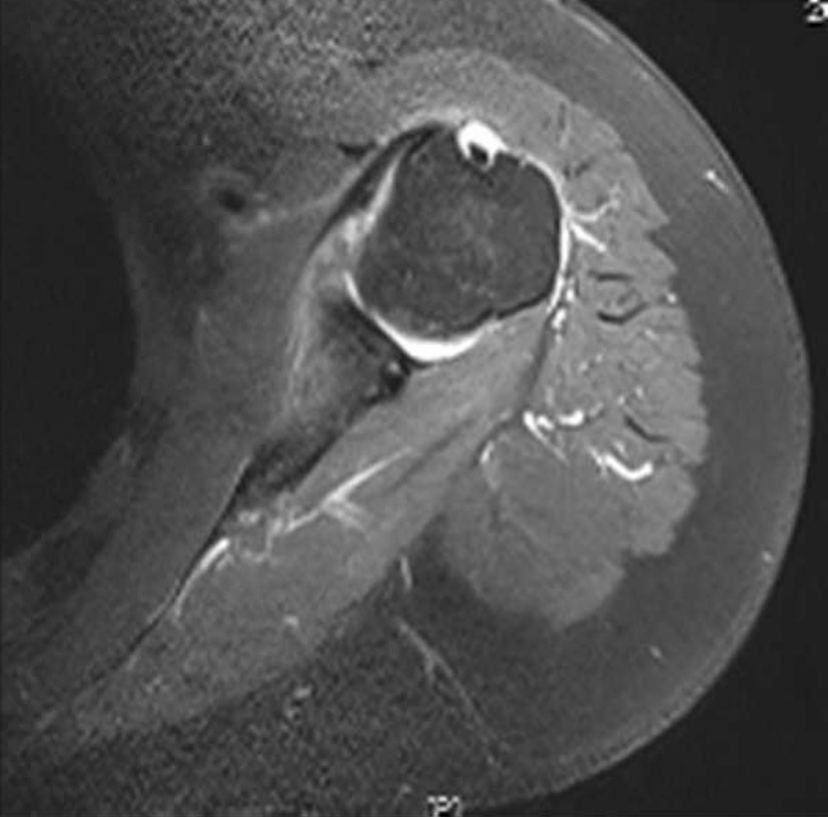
T2-weighted magnetic resonance (MRI) axial view of a diseased biceps tendon
